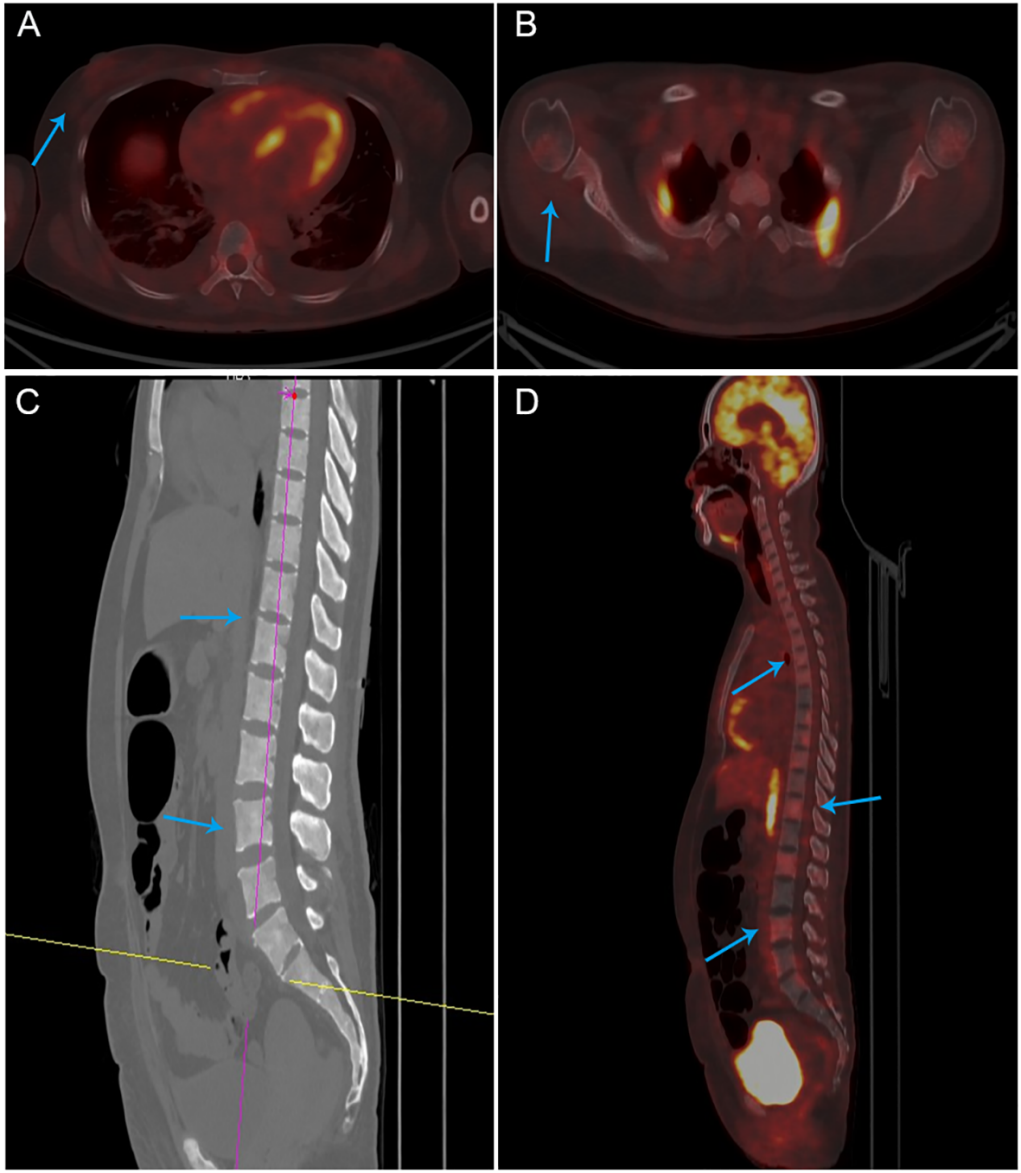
PET-CT images (A, B) There was no abnormal metabolism in the area of the right breast surgery or the axilla. (C, D) Diffuse hyperdensity in the medullary cavity of the bone, no destruction of the bone cortex.
